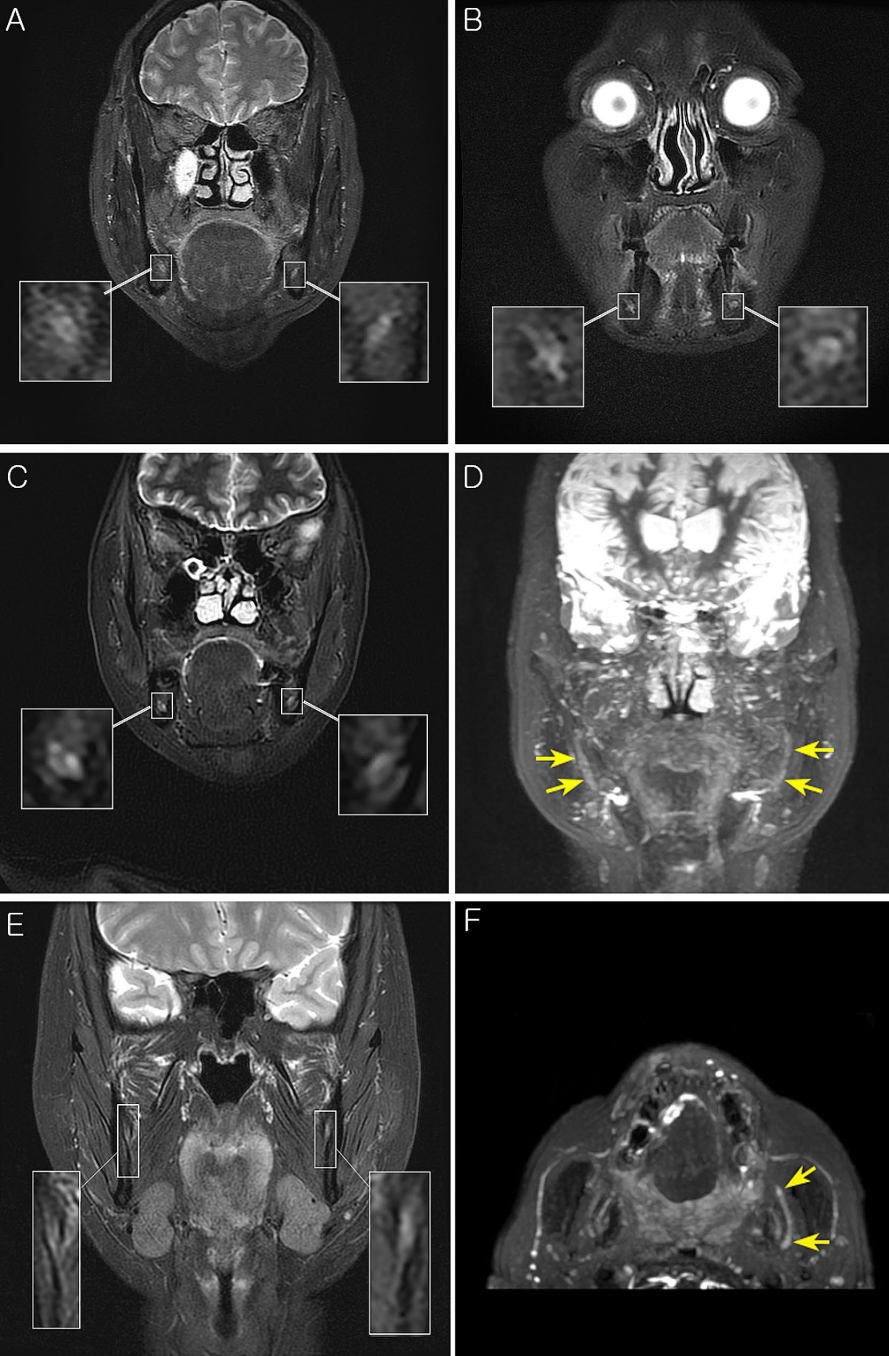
Example MR images demonstrating features of present study. (A-B) Representative high contrast MR images with sufficient resolution to demonstrate nerve fascicles; (C) Representative cross-sectional view demonstrating higher signal of trauma side (Right IAN); (D) Maximum intensity projection of (C) also demonstrates asymmetric hyperintensity of traumatized (right) nerve; (E) Representative cross-sectional T2 Flex image including the ROI of the traumatic left lingual nerve; (F) Maximum intensity projection of (E) demonstrating ‘Triple B’ sign of left lingual nerve (arrow)
